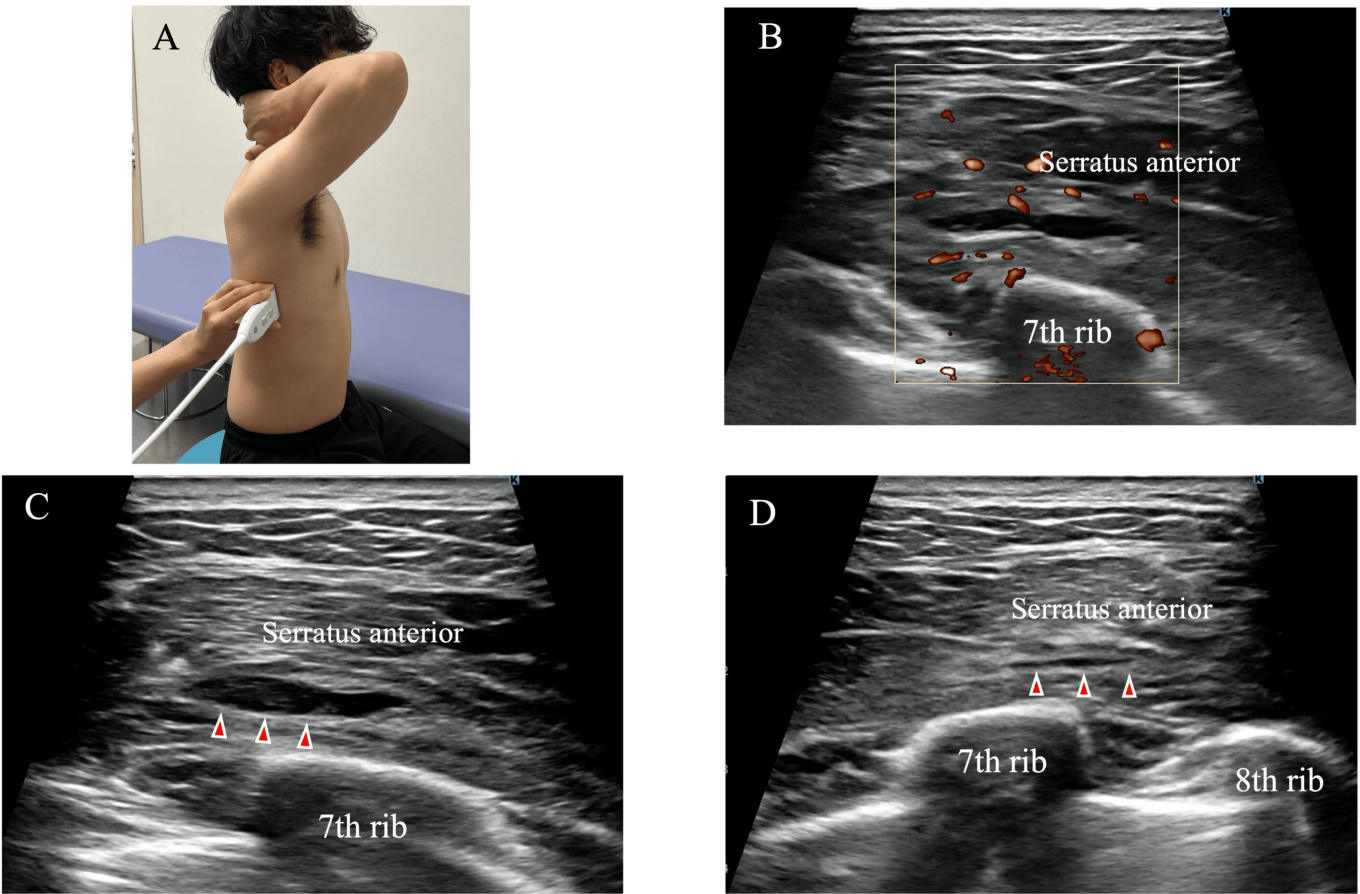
Ultrasonographic findings at the injury site (A) Patient positioned to visualize the lower fibers of the serratus anterior muscle. (B) Color Doppler imaging showing increased vascular signals around the hypoechoic area. (C) Hypoechoic area clearly visible without probe compression. (D) Hypoechoic area decreased in size under manual probe compression, suggesting a compressible lesion such as a hematoma.
